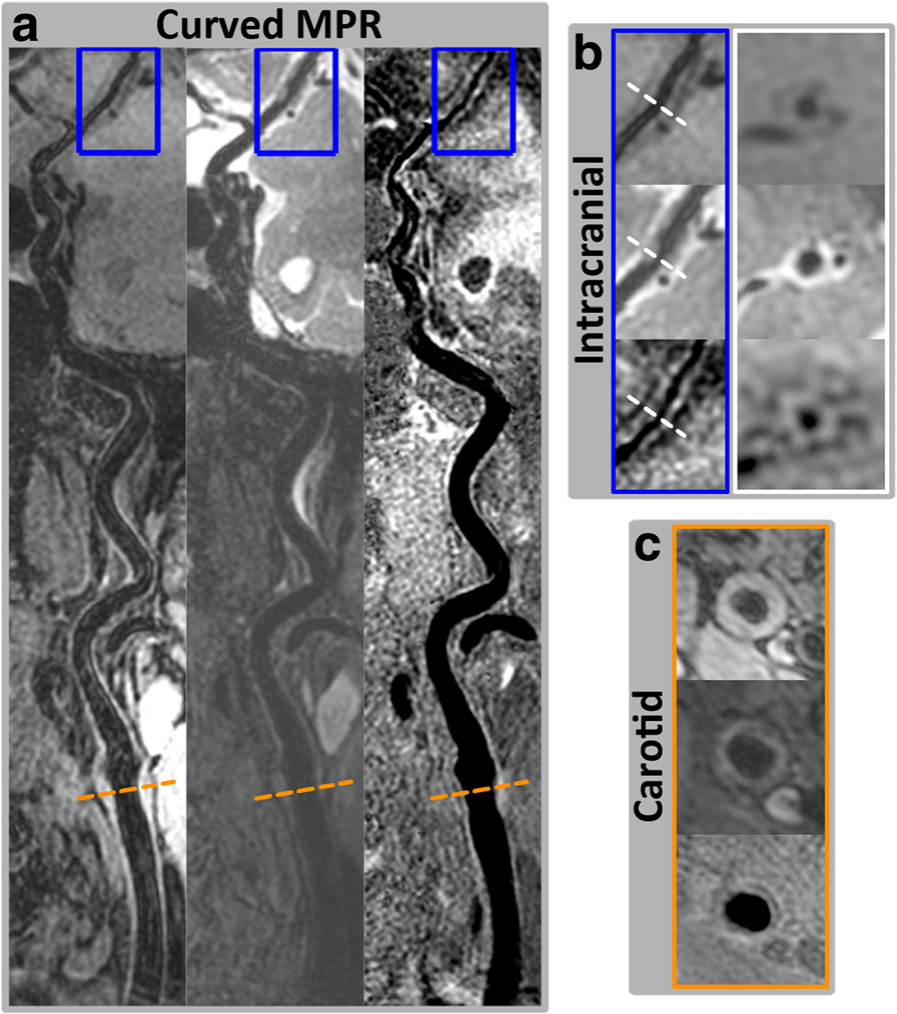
Example images showing the capability of arbitrary view for intra- and extracranial vessel wall delineation. An overview of vessel wall across multiple arteries can be provided using 3D isotropic VWI and curved MPR technique (a). Note that vessel wall thickening at the intracranial MCA M1 (b) and carotid bifurcation (c) can be clearly identified with 3D-MERGE, T2-weighted VISTA and SNAP sequences
